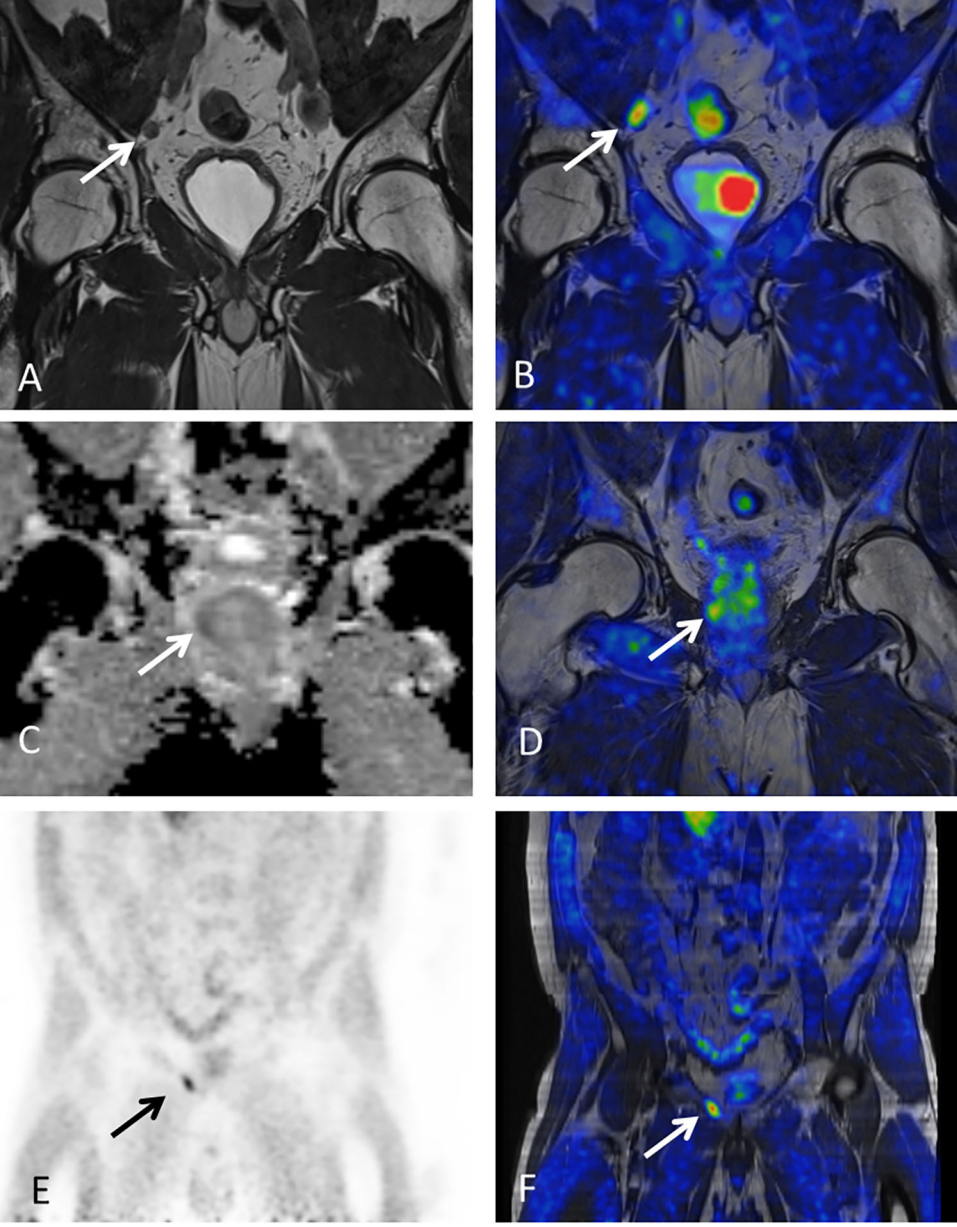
Examples of lesion visibility. Arrows point out lesions detected by ^18^F-fluciclovine PET/MRI. (A, B) 57-year-old patient with PSA of 0.6 ng/mL and one pelvic lymph node suspicious for metastasis based on mpMRI (A) and PET (maximum standardized uptake value [SUV max]=3.95) (B). (C, D) Prostatic lesion with restricted diffusion [Apparent diffusion coefficient (ADC)=982 x10^−6^mm^2^/s] (C) and increased tracer uptake (SUVmax=4.19) (D) in 62-year-old man with history of primary EBRT and PSA of 5.5 ng/mL at time of imaging. (E, F) Pelvic bone lesion (SUVmax=4.04) in 60-year-old male with PSA of 1.7 ng/ml. This lesion was initially missed by mpMRI but could be detected retrospectively.
